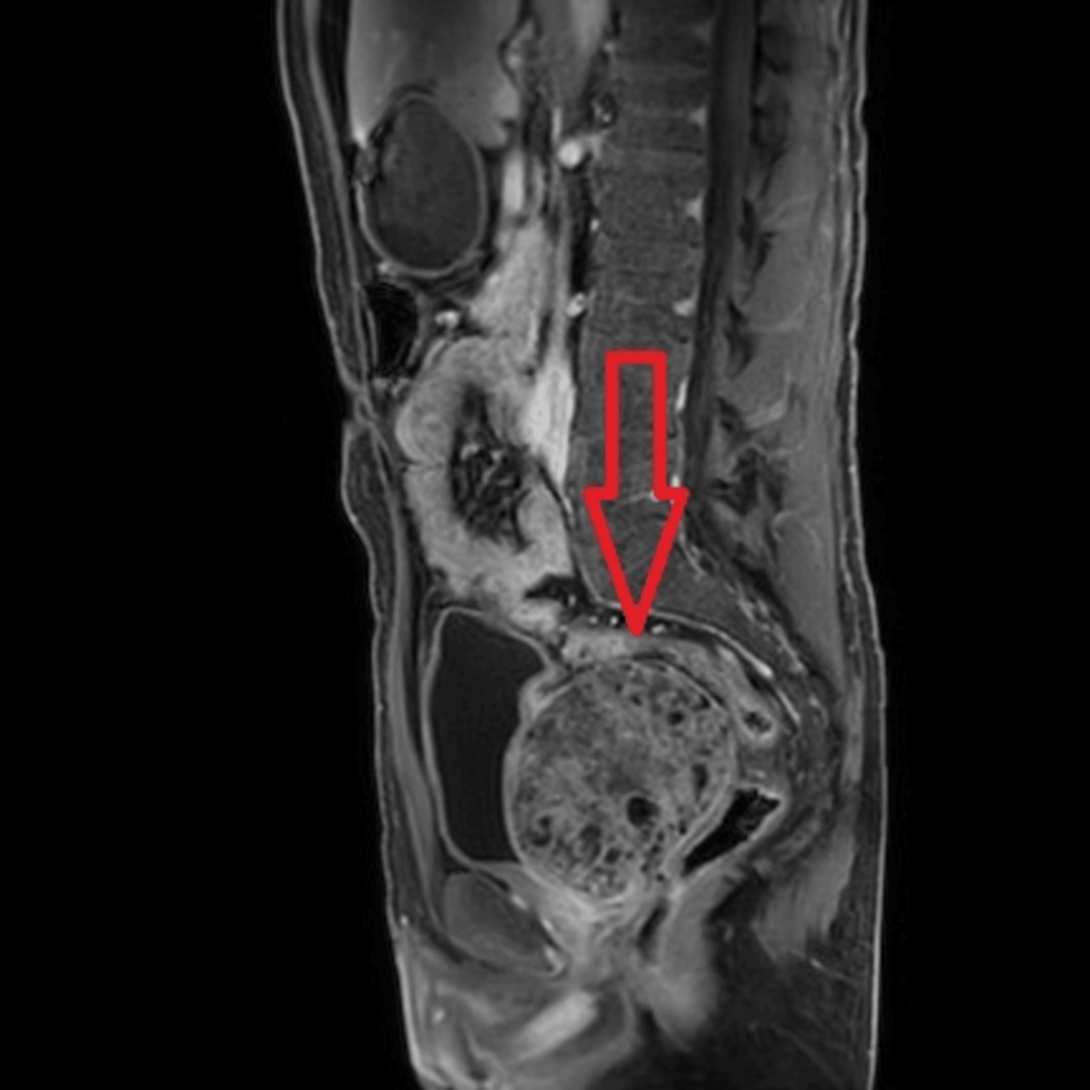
Abdominal MRI scan using the mDIXON method in sagittal plane showing sizable pelvic mass (arrow).
